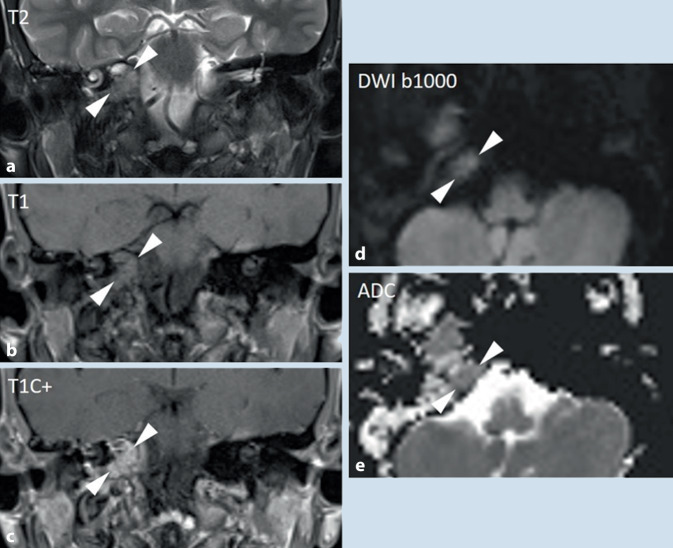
a T2-gewichtet zeigt sich eine geringe Hyperintensität des petrösen Apex mit isointensem Signal in b T1 sowie homogenem Kontrastmittelenhancement in c T1C+. Es besteht keine Diffusionsstörung bei nur geringer Hyperintensität in der d DWI (Folge des T2-Shine-Through-Effekts) und ohne relevanten Abfall der e Apparent Diffusion Coefficient-Werte (ADC).
